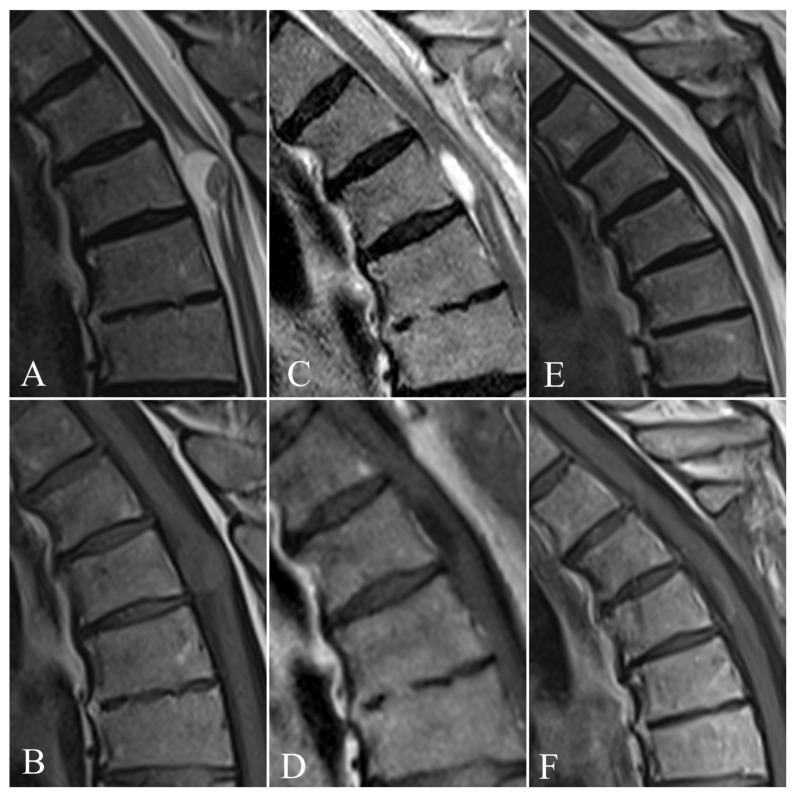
Magnetic resonance imaging (MRI) showing the sagittal scans: (A) T2-weighted image and (B) T1-weighted images of an expansile intramedullary solid-cystic lesion measuring 16 mm × 11 mm × 10 mm, without contrast enhancement, at the T4 level, with segmental widening of the spinal cord above and below the lesion. The early follow-up MRI shows no pathological mass and postoperative changes on both (C) T1-weighted and (D) T2-weighted images. The MRI performed 6 months after surgery revealed complete abscess excision in (E) T2-weighted and (F) T1-weighted sequences.
